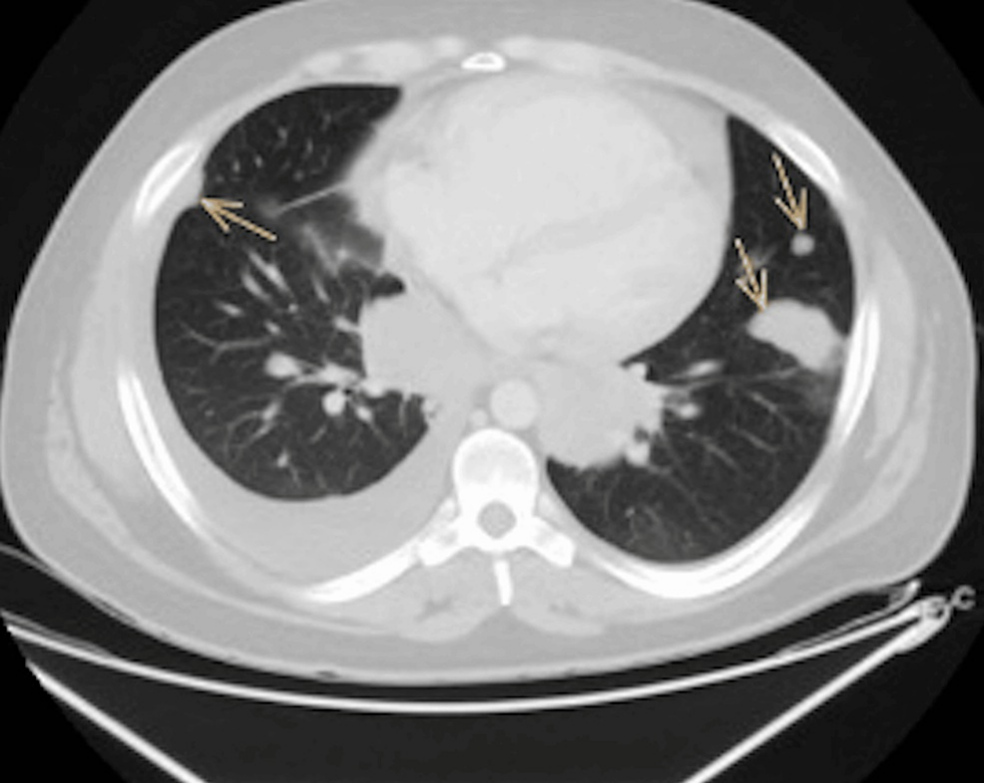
Axial CT chest with IV contrast study showed pulmonary parenchymal and pleural-based soft tissue metastasis. There is a pleural fluid collection on the right side. Computed tomography (CT); Intravenous (IV)
